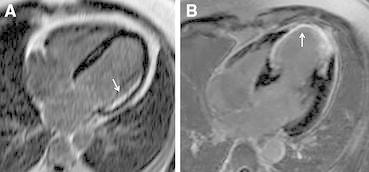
Contrast-enhanced magnetic resonance imaging for characterization of myocardial scar. Selected 4-chamber views on contrast-enhanced MRI of two patients with ischemic cardiomyopathy show non-transmural subendocardial scar (A
